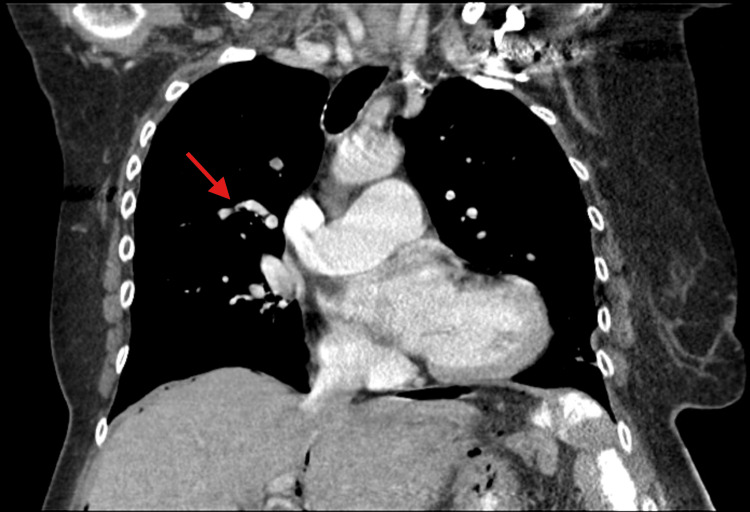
Pulmonary embolism identified on admission CT chest/abdomen/pelvis (CT C/A/P); the arrow indicates a filling defect.
